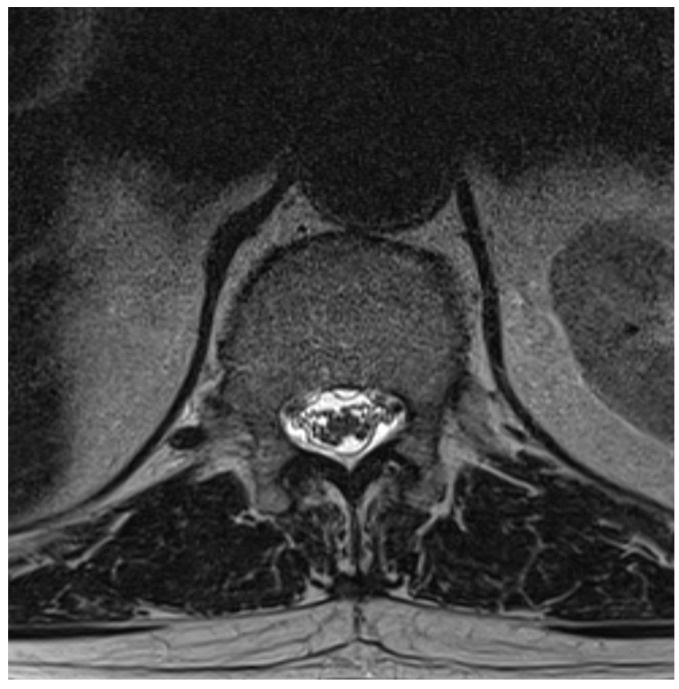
Axial T2-weighted MRI at L1, showing epidural cerebrospinal fluid accumulation both ventral and dorsal to the thecal sac.
